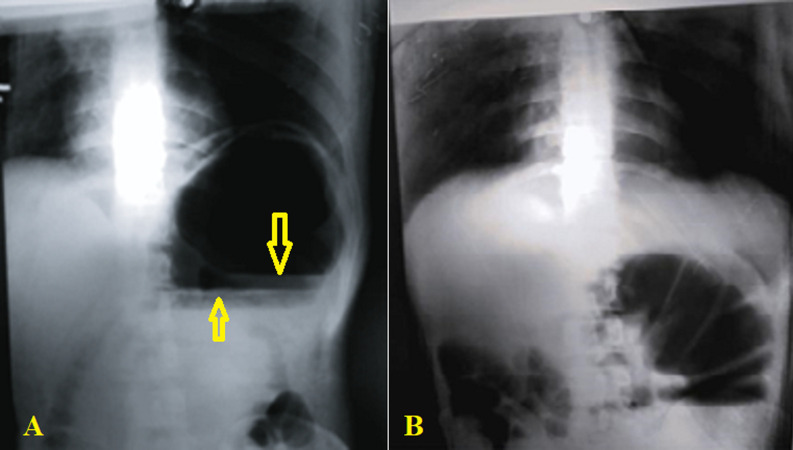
plain abdominal radiography showing two large well-defined air-fluid levels approximately 15 cm in width superimposed on each other located at the left upper quadrant of the abdomen (arrows), with minimal gas seen at the distal bowel loops; A) erect view; B) supine view
